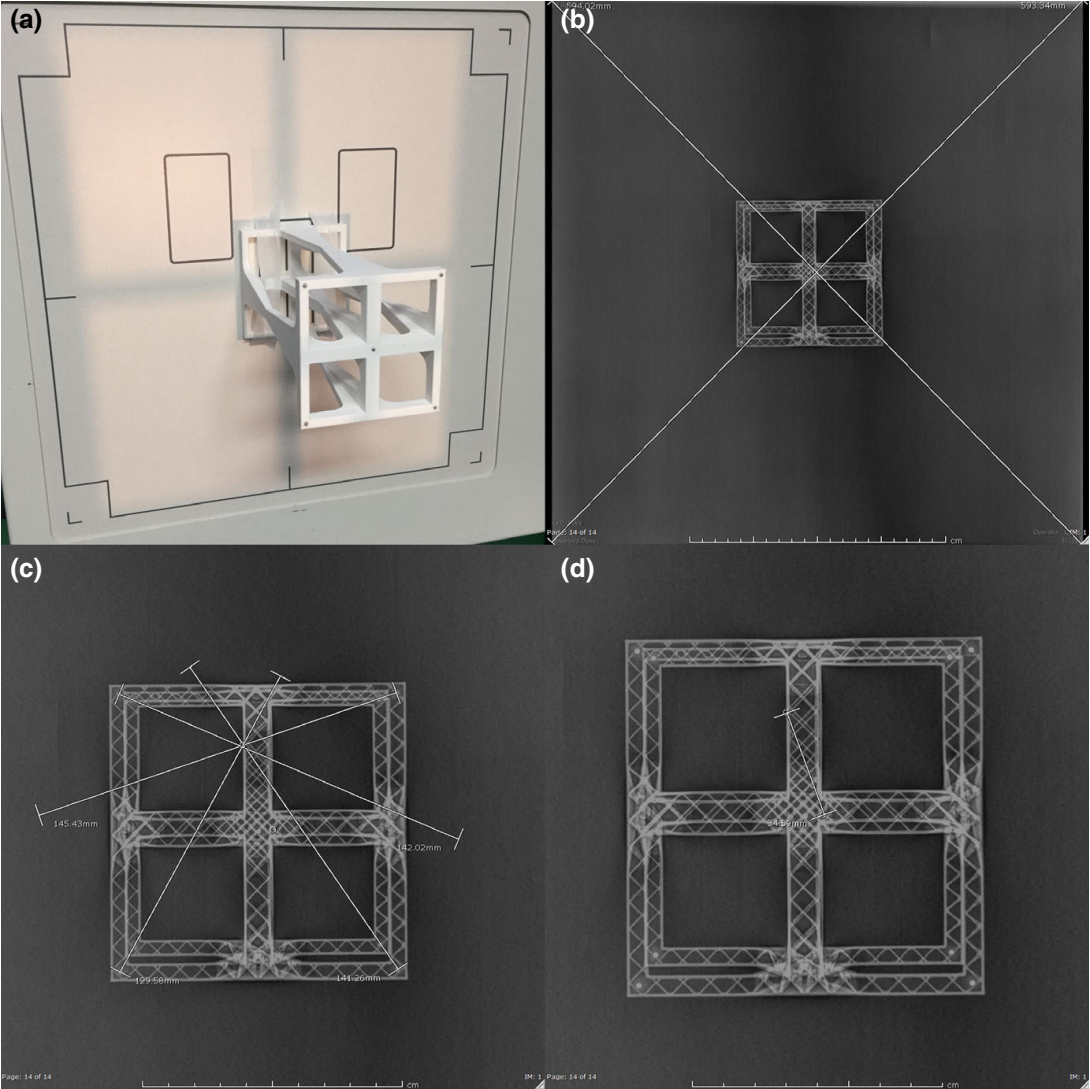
Perpendicularity test tool. Images show (a) the test tool taped to an upright image receptor, (b) the resulting radiograph acquired with a flat panel detector with diagonal lines indicating the center of the image receptor, (c) the fiducial markers connected by lines drawn on a PACS workstation showing the location of the perpendicular ray, and (d) a final measurement of the distance between the perpendicular ray and the detector center. The intersections were marked with letters “O” and “C” using the annotation tool on the PACS workstation.
